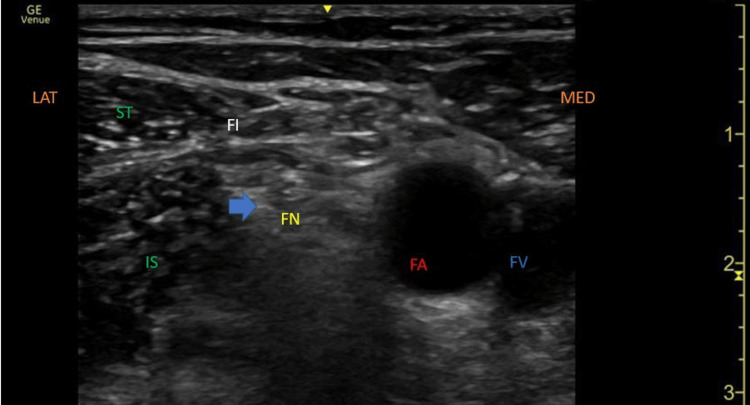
Ultrasound image using a linear array transducer demonstrating the anatomy of the femoral triangle with final lead placement FA, femoral artery; FV, femoral vein; FN, femoral nerve; IS, Iliopsoas muscle; ST, sartorius muscle; FI, fascia lliaca; arrow, final stimulating lead position; MED, medial; LAT, lateral
